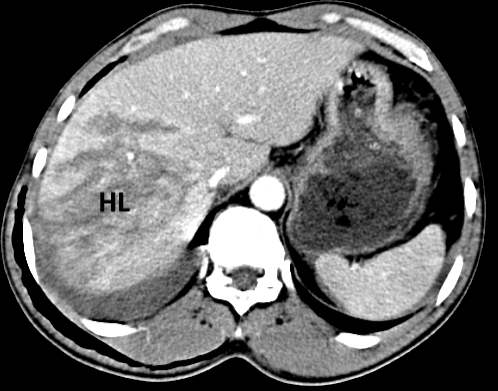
Abdominal CT. Degree Ⅳ liver laceration.
